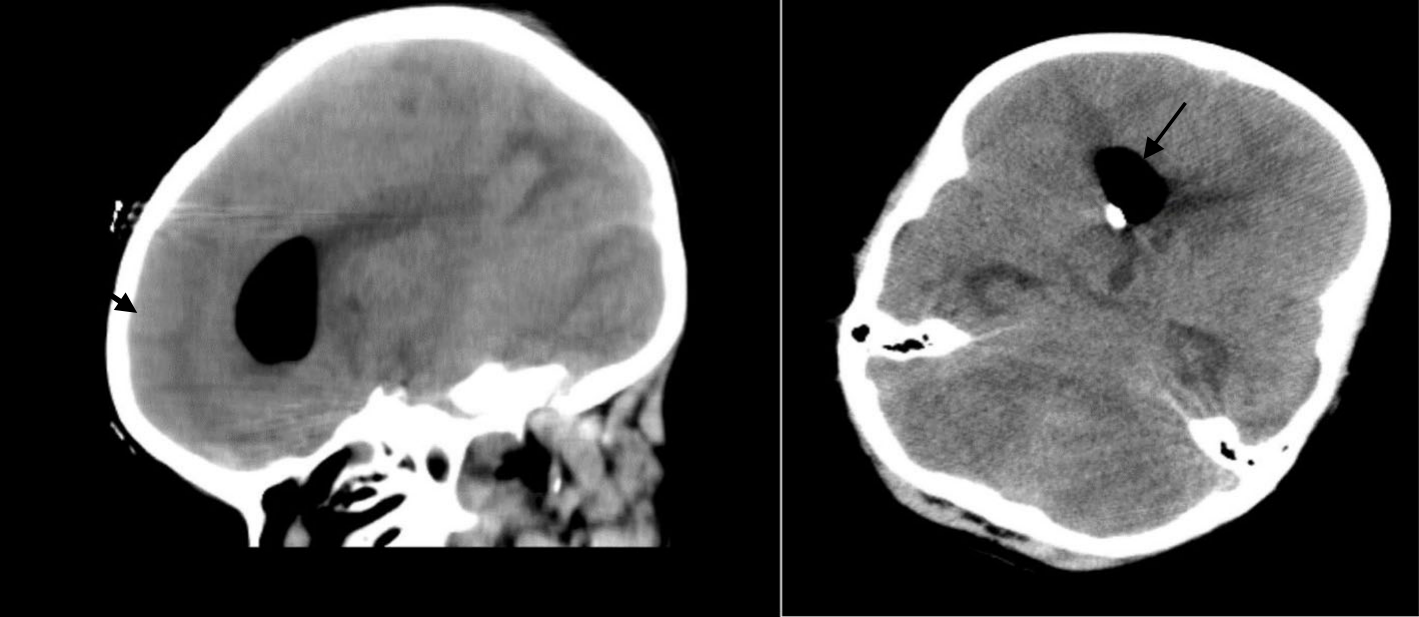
CT images of a 4-year-old patient who underwent Fontan surgery in single ventricular physiology after postoperative bleeding in the left temporal lobe (indicated by the black arrow). Extensive intracranial bleeding developed in the patient’s left temporal region on the fourth postoperative day after Fontan surgery. A drainage set was inserted by neurosurgery to relieve signs of compression
